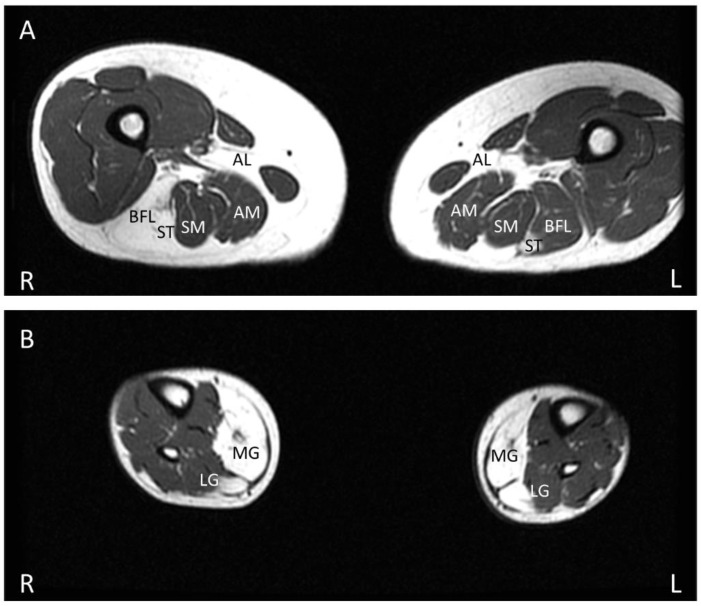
T1-weighted lower limb MRI in a patient with LGMDR12, calf atrophy and longstanding history of exercise intolerance and rhabdomyolysis showing asymmetrical fatty replacement of predominantly posterior lower limb compartment muscles, including bilateral adductor longus, long head of right biceps femoris, and bilateral semitendinosus (A) and bilateral medial gastrocnemius and parts of lateral gastrocnemius (B). AL—adductor longus, AM—adductor magnus, SM—semimembranosus, ST—semitendinosus, BFL—long head of biceps femoris, MG—medial gastrocnemius, LG—lateral gastrocnemius.
